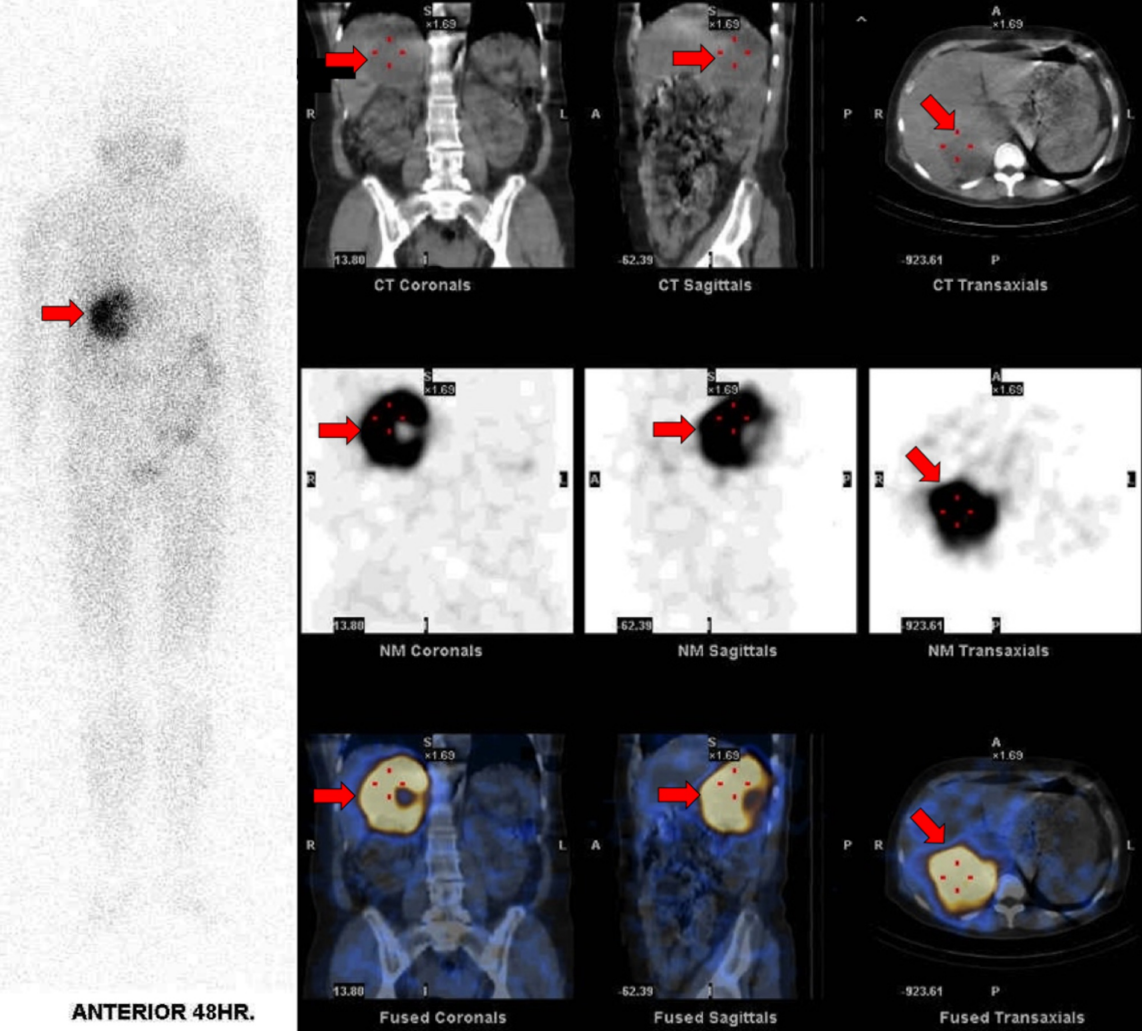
Meta-iodobenzylguanidine (MIBG) single photon emission computed tomography (SPECT/CT) MIBG SPECT/CT demonstrates avid unilateral radiotracer uptake in the large right adrenal mass (arrow), which is consistent with pheochromocytoma.
